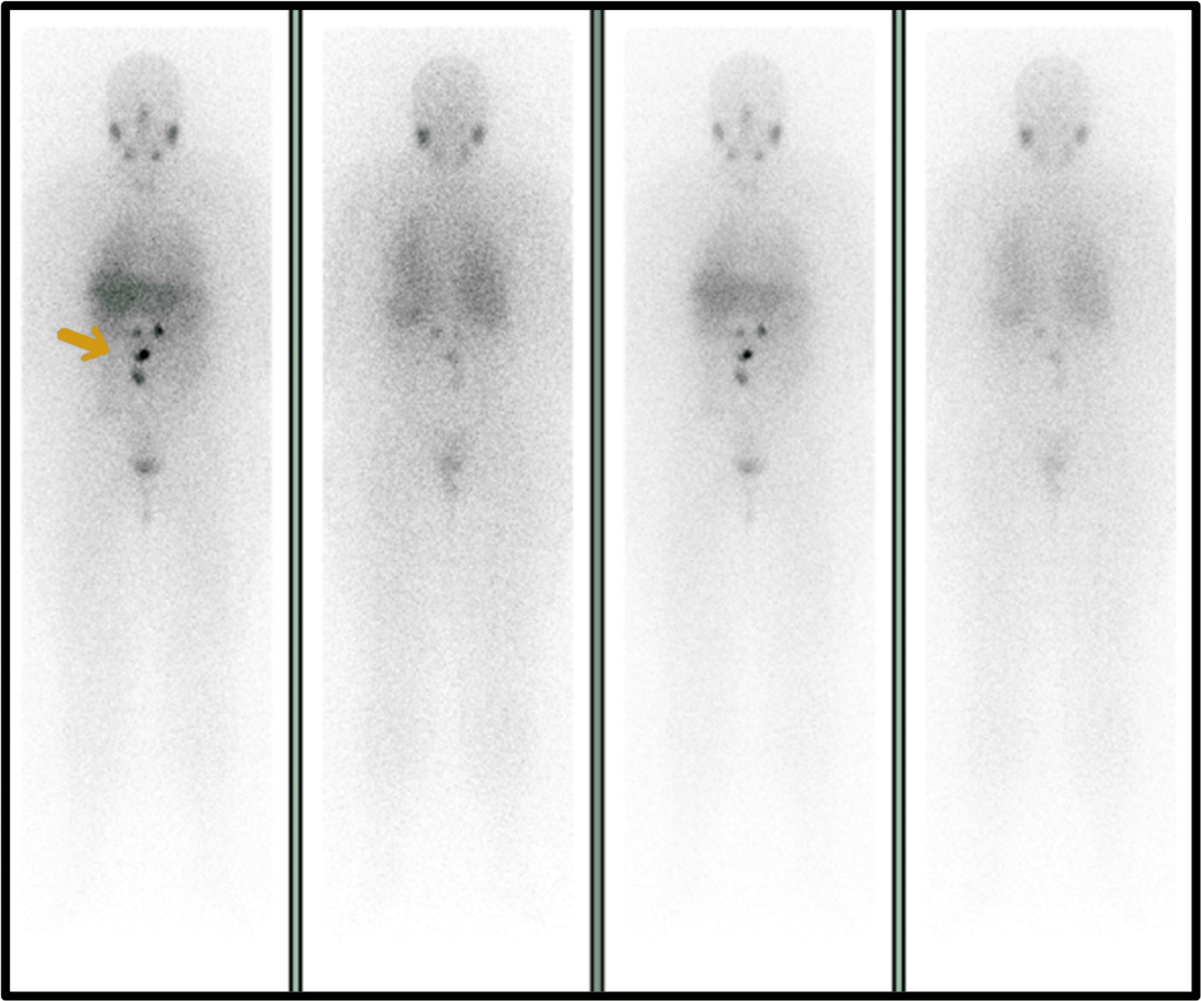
Postoperative scintigraphy showing abnormal ¹²³I-MIBG uptake Postoperative scintigraphy revealed four foci of abnormal ¹²³I-MIBG uptake: two in the epigastric/mesogastric transition and two in the mesogastric region.
